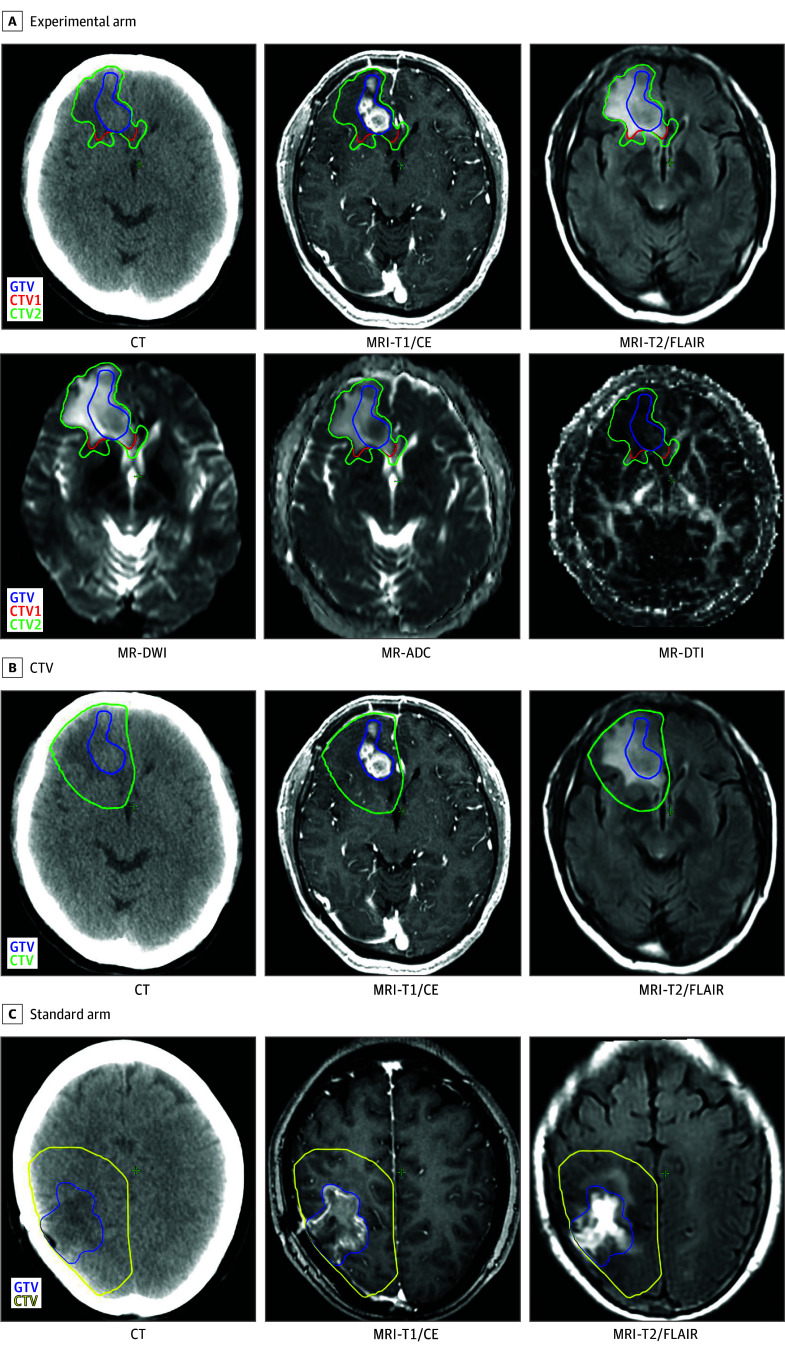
Target Volume Definition A case of right frontal glioblastoma (experimental arm) (A) with the clinical target volume (CTV) (yellow contour) delineated according to the approach of a case or right occipitoparietal glioblastoma (standard arm) (C). ADC indicates apparent diffusion coefficient; CT, computed tomography; DTI, diffusion tensor imaging; DWI, diffusion-weighted imaging; GTV, gross tumor volume; MR, magnetic resonance; MRI, magnetic resonance imaging; T1/CE, T1 weighted and contrast enhanced; T2/FLAIR, T2 weighted and fluid-attenuated inversion recovery.
